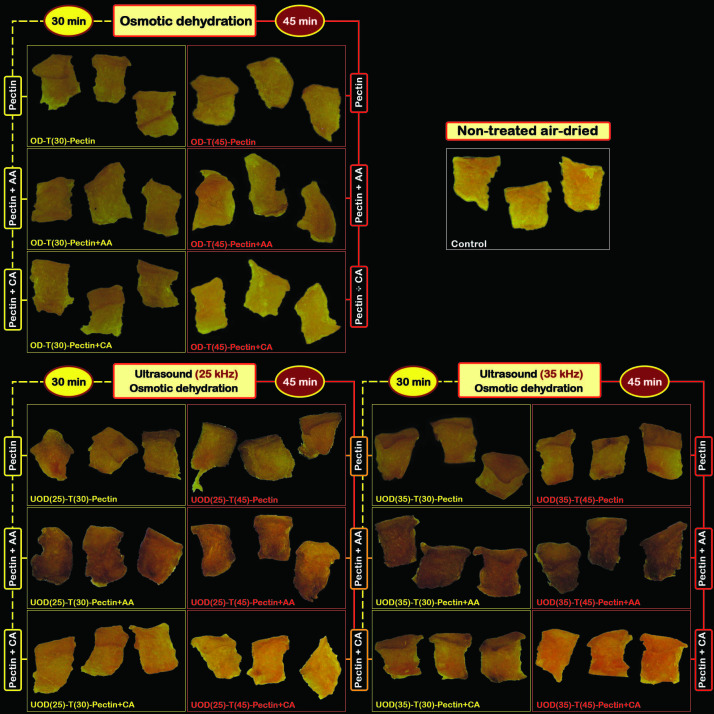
Images of non-treated apricots (control) and samples treated by osmotic dehydration (OD) for 30 and 45 min (T30 and T45), ultrasound-assisted osmotic dehydration (UOD) at frequencies of 25 and 35 kHz for 30 and 45 min (T30 and T45), followed by the application of pectin, pectin with citric acid (CA), and pectin with ascorbic acid (AA) coatings
